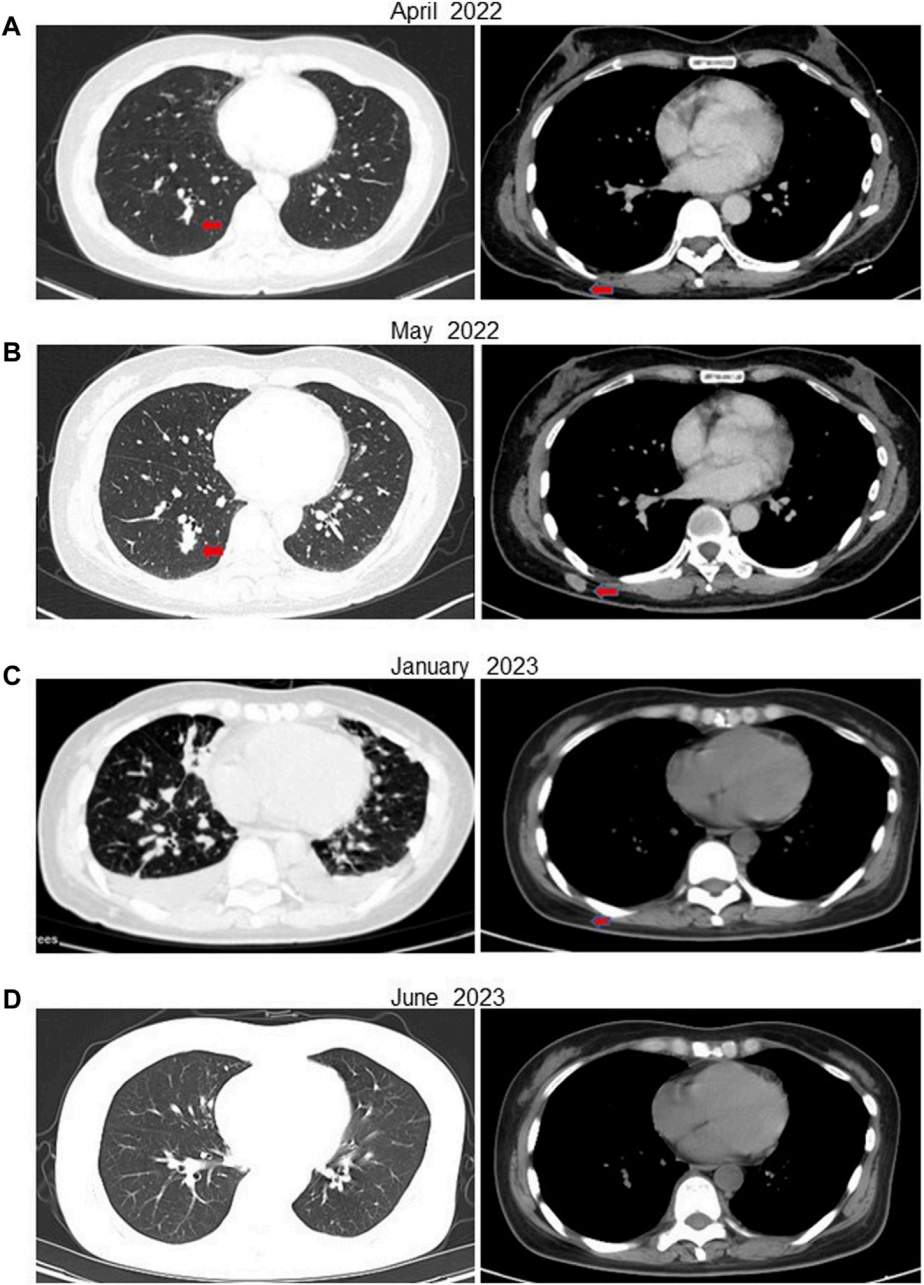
CT of the patient at various stages after receiving treatment. (A) In April 2022, after 6 weeks of treatment with pembrolizumab in combination with sunitinib, the patient’s metastases were significantly reduced. (B) In May 2022, after 5 weeks of treatment with pembrolizumab in combination with axitinib due to the patient’s intolerance of sunitinib, the subcutaneous nodule on the right side of the back was enlarged compared with the previous one. (C) After eight cycles of the original regimen, a CT in January 2023 showed multiple metastases in both lungs that were significantly more advanced than before. (D) ACT in July 2023 showed a significant reduction in the patient’s metastatic lesions.
